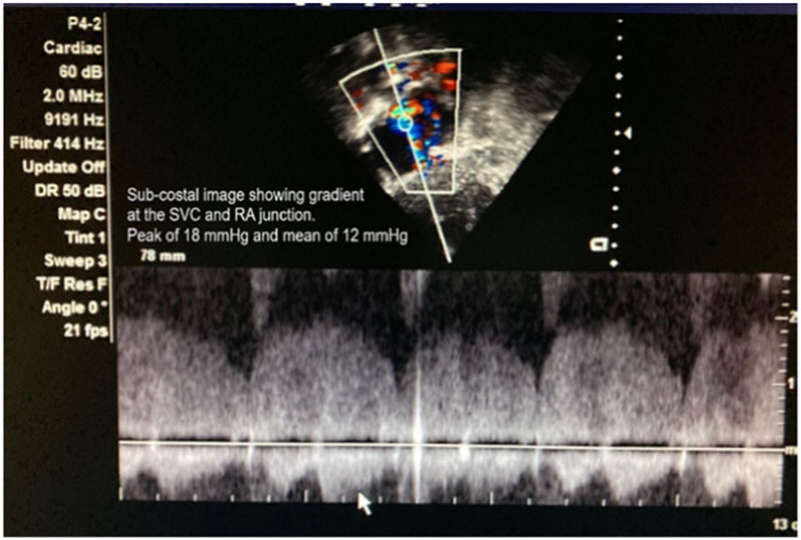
Postoperative two‐dimensional echocardiogram for patient P2. RA, right atrium; SVC, superior vena cava (Image credits: Dr. Bhadra Y. Trivedi).
